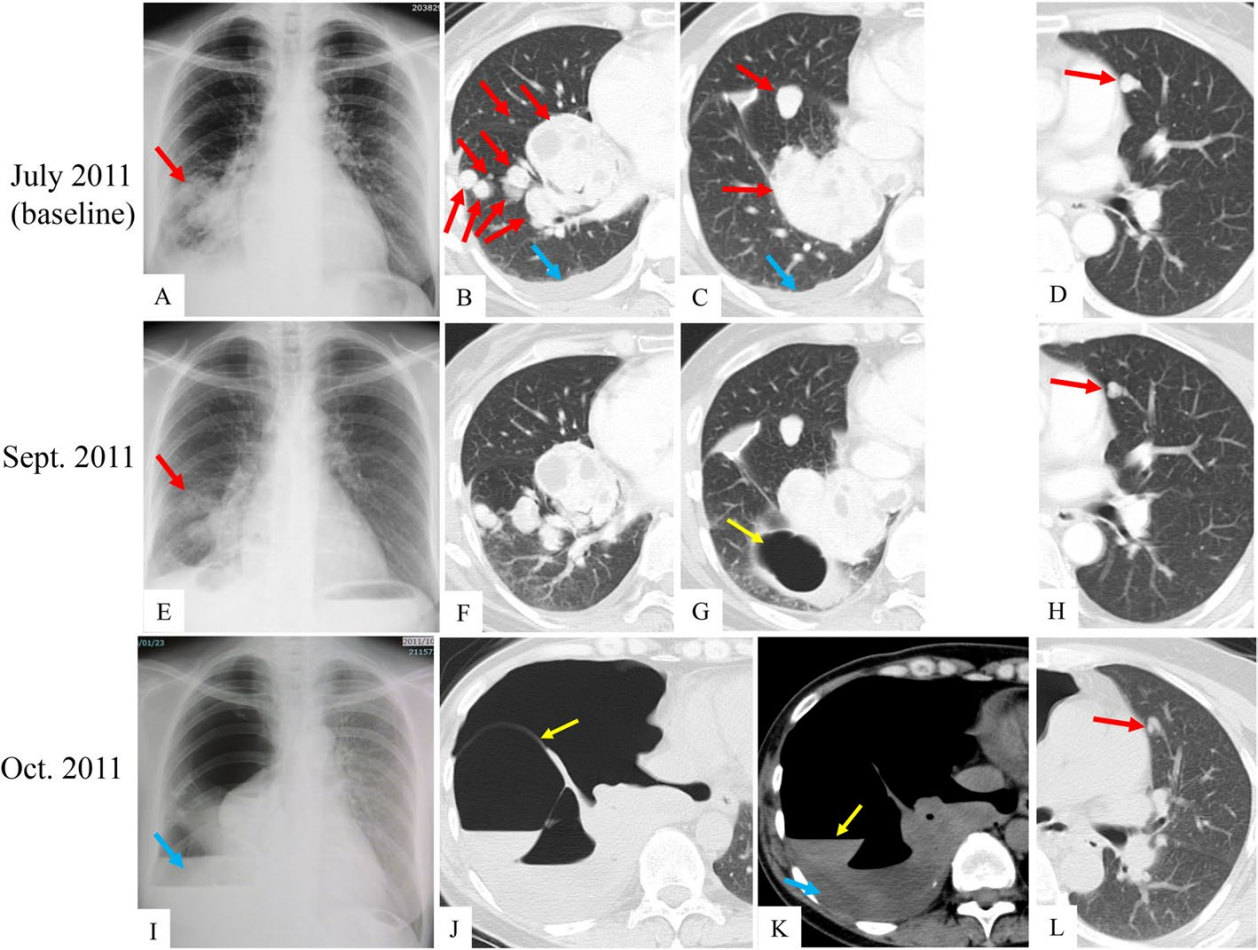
Representative chest radiography and CT images at baseline (A–D), during chemotherapy (E–H), and after the onset of pneumothorax with mediastinal shift to the contralateral side (I–L). Red arrows indicate metastatic tumors; blue arrows, right pleural effusion; yellow arrows, a giant cyst that appeared in the bottom of the basal segment during treatment
